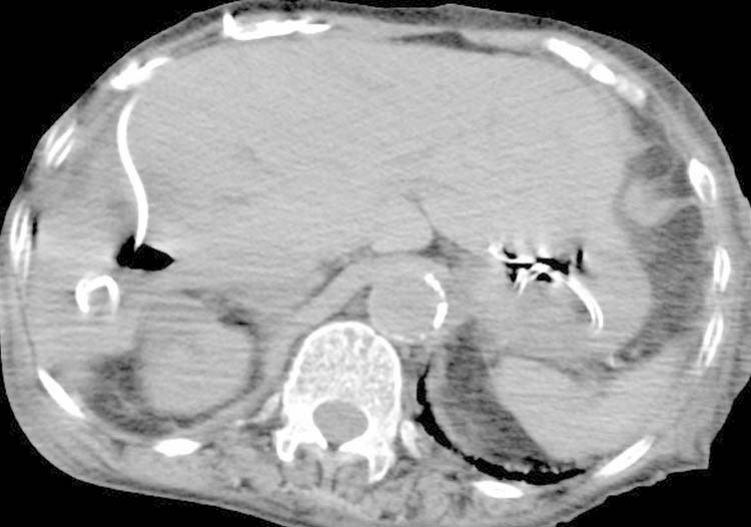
Abdominal CT shows a reduction in the size of the hepatic cyst infection.
